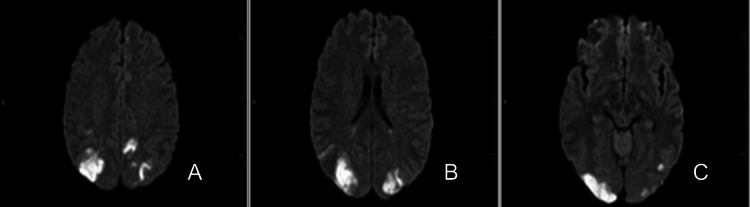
MRI brain (diffusion-weighted imaging (DWI) series, axial sections) demonstrating acute/subacute multifocal infarcts in a multi-arterial territory distribution, including the (A) bilateral parietal lobes and (B, C) bilateral occipital lobes. These findings are suggestive of reversible cerebral vasoconstriction syndrome (RCVS), a condition that can lead to cerebral ischemia due to the transient narrowing of multiple cerebral arteries. The infarcts observed in distinct arterial territories are a common manifestation of RCVS, which is often associated with sudden-onset headaches and can result in neurological deficits, including vision impairments, as seen in this patient.
